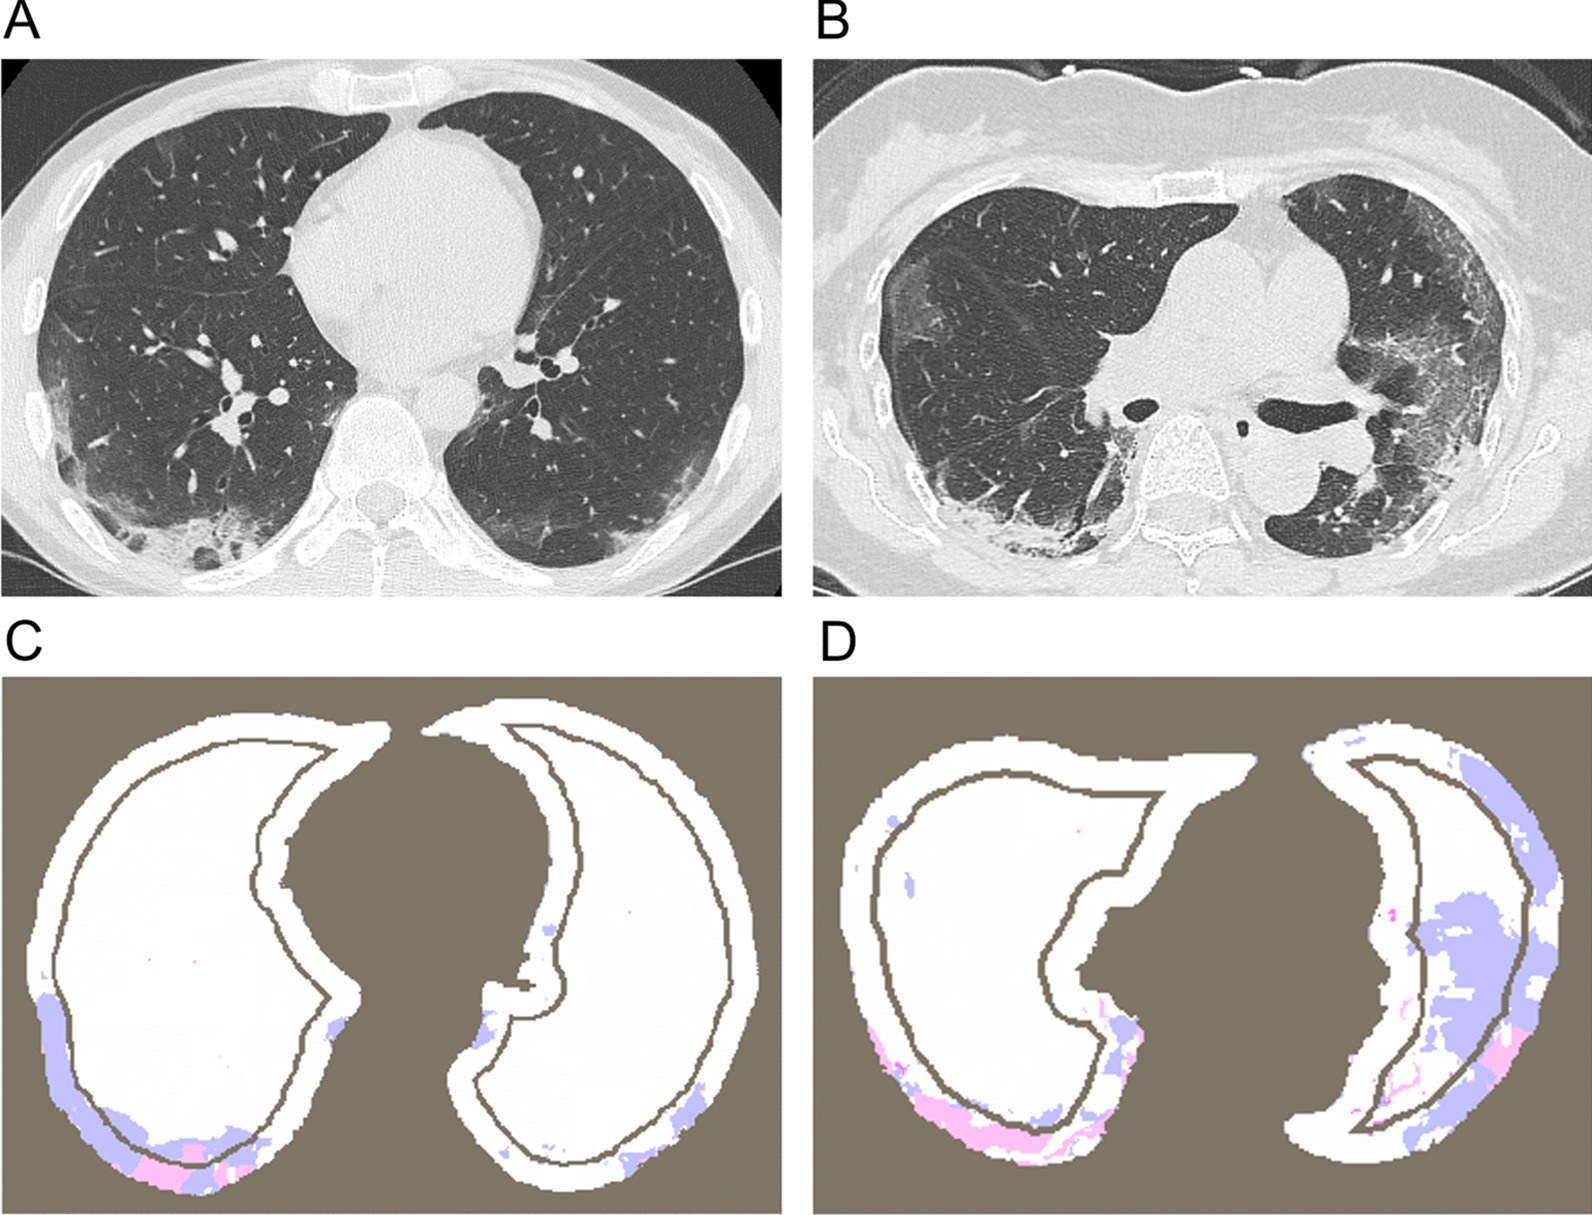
Representative images of SARS-CoV-2 pneumonia. A and B indicate two representative CT images of the nonsevere and severe cases, respectively. C and D are corresponding images after segmentation of lungs, ground-glass opacification/opacity (GGO) and consolidation (CON). The blue and pink colored areas indicate the area of GGO and CON respectively. In the nonsevere case (A, C), the percentages of whole, peripheral, and central lungs occupied by GGO (GGO%, GGO%(P), GGO%(C)) were 5.61%, 11.0%, and 2.22%, respectively. The percentage of whole, peripheral, and central lungs occupied by CON (CON%, CON%(P), CON%(C)) were 5.37%, 3.54%, and 6.52%, respectively. In the severe case (B, D), GGO%, GGO%(P), GGO%(C) were 9.68%, 10.8%, 8.31%, and CON%, CON%(P), CON%(C) were 12.1%, 15.0%, 8.70%
